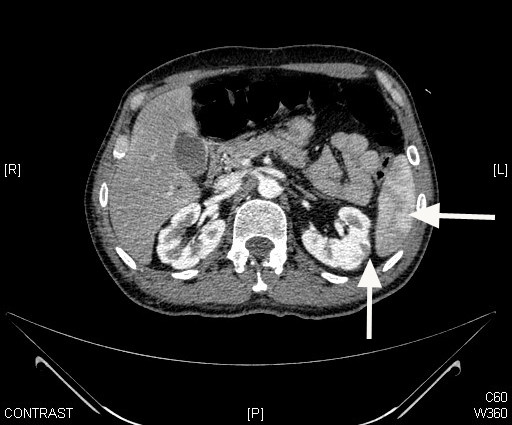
Axial CT scan showing infarcts in the spleen (right arrow) and the left kidney (left arrow).
